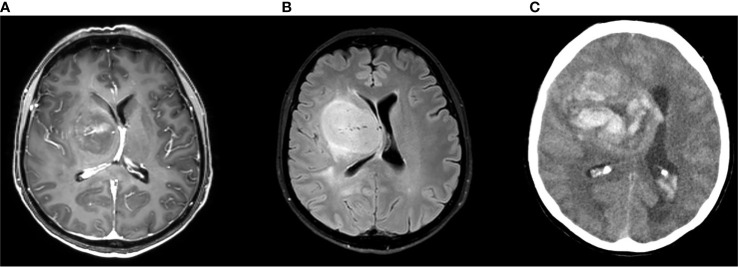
Brain MRI scan (A: gadolinium enhanced image B: FLAIR image) of a 53-year-old woman complaining of subacute onset of mild left hemiparesis showing highly vascularized HGG; 8 days later the patient developed headache and brain CT scan (C) showed a wide intralesional bleeding.
